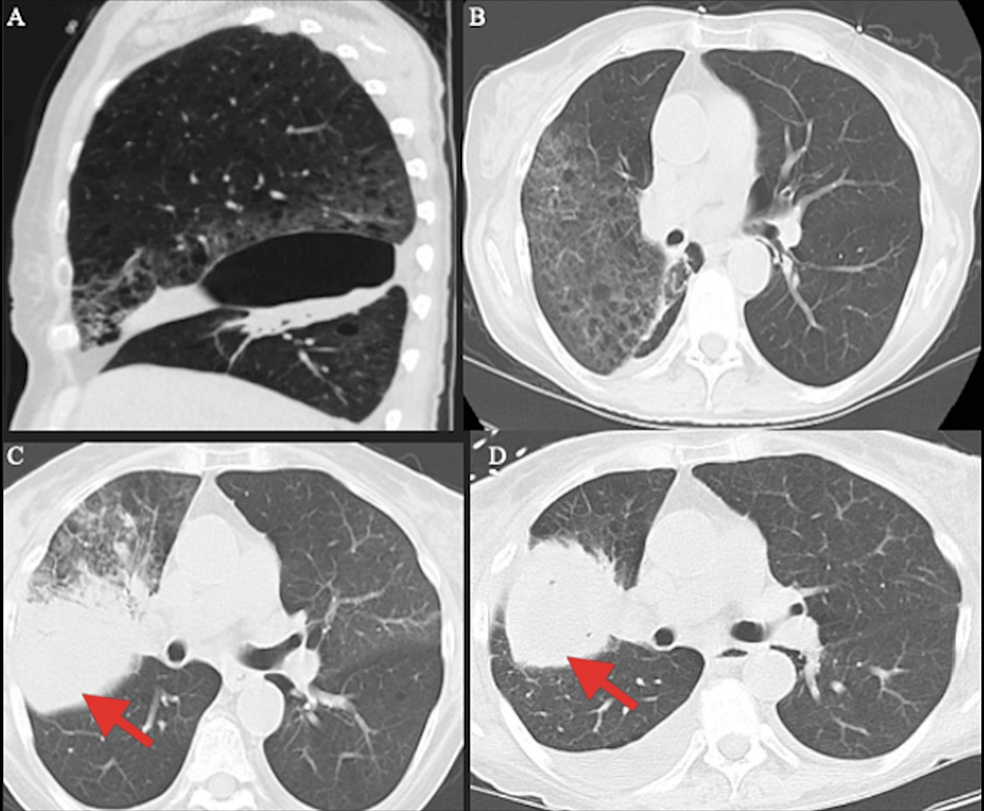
Computed tomography imaging findings over three hospital admissions A) First admission: complete consolidation of the right middle lobe. Right upper lobe (RUL) with a ground glass appearance. Large oval air bulla along the right major fissure. B) First admission: ground glass appearances. C) Second admission: increased consolidative changes in the RUL and small lung pleural effusion. D) Third admission: mass-like consolidation in RUL and increased size of right-sided effusion.
